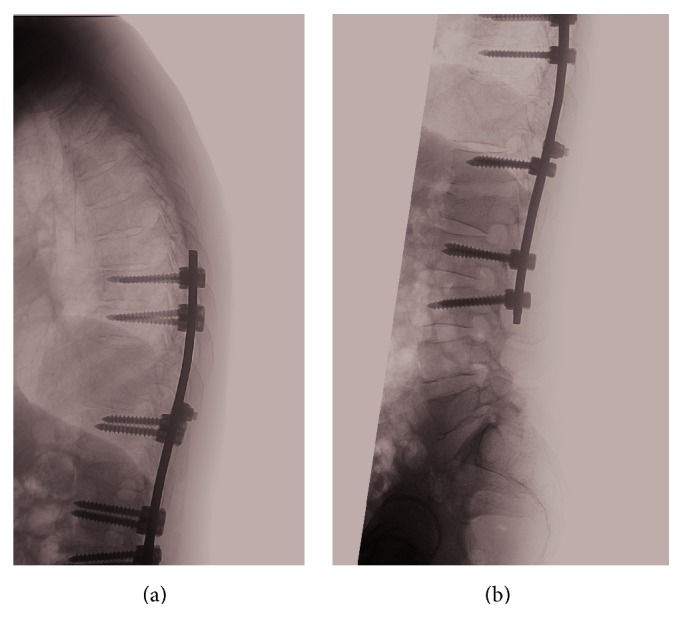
Postoperative plain radiograph showing segmental instrumentation from T8 to L3 vertebrae.
